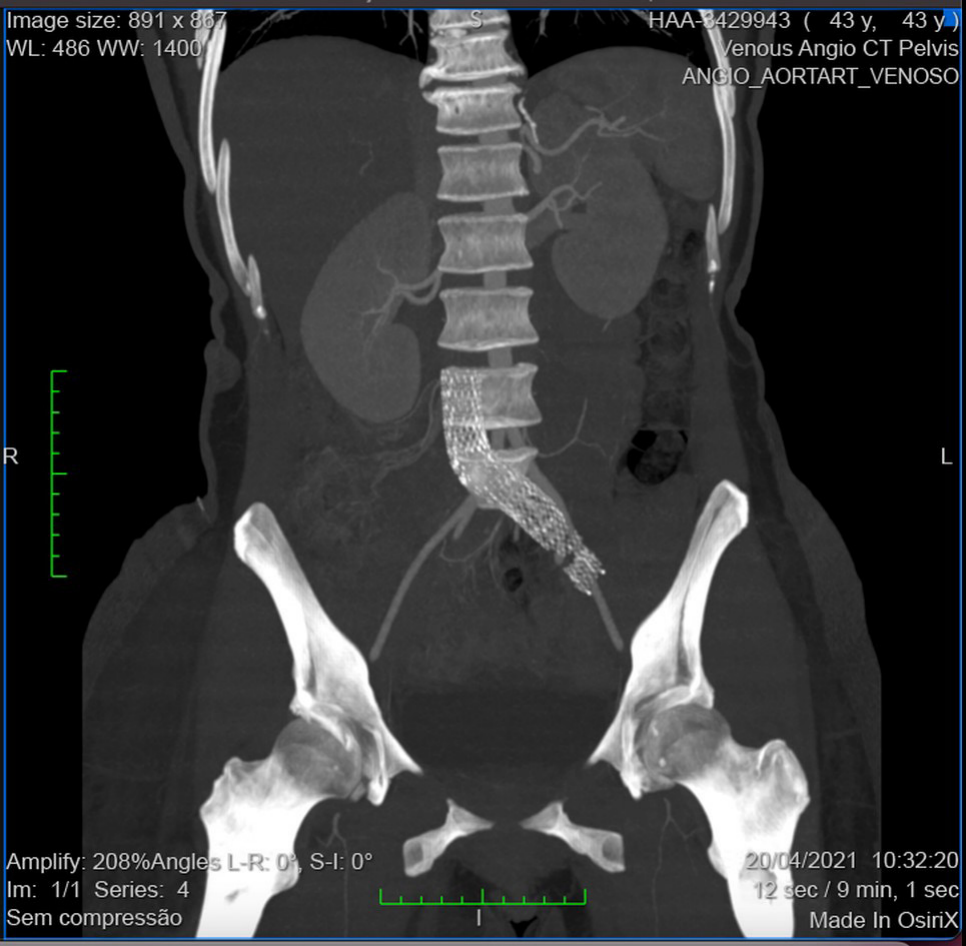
Computed tomography angiography showing the venous stent in the inferior vena cava and left common iliac vein.
